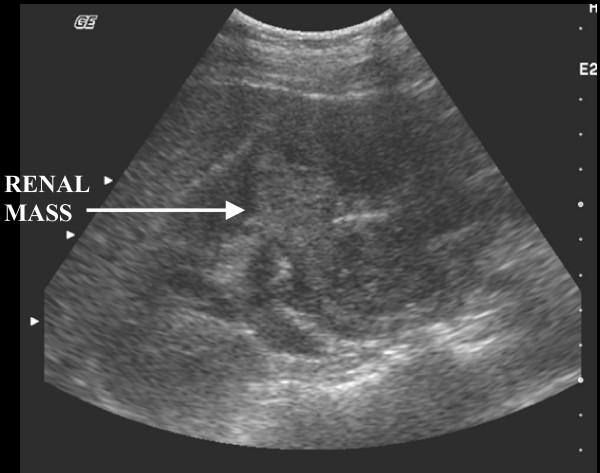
This is a picture of renal ultrasound. It shows echo-dense area in the renal area, suggestive of a renal tumour.
